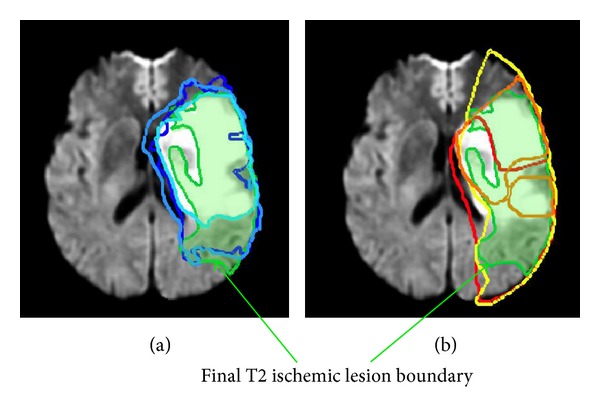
Time-indexed penumbra and core lesion boundaries combined to final T2-weighted boundary imaged at >1 month. (b) MTT manually delineated penumbra boundaries consecutively at *t*
